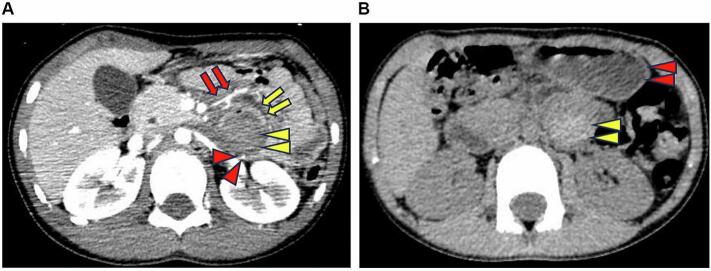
A: Axial contrast-enhanced computed tomography scan performed on the day of injury showed a mesenteric hematoma (yellow arrowhead) compressing the third portion of the duodenum (yellow arrow), the jejunal artery around the hematoma (red arrow), and the inferior mesenteric vein running dorsal to the hematoma (red arrowhead). B: Axial plain computed tomography scan performed on the 10th after the injury showed a mesenteric hematoma of the same size (yellow arrowhead) and gastric dilatation (red arrowhead). (For interpretation of the references to colour in this figure legend, the reader is referred to the web version of this article.)
